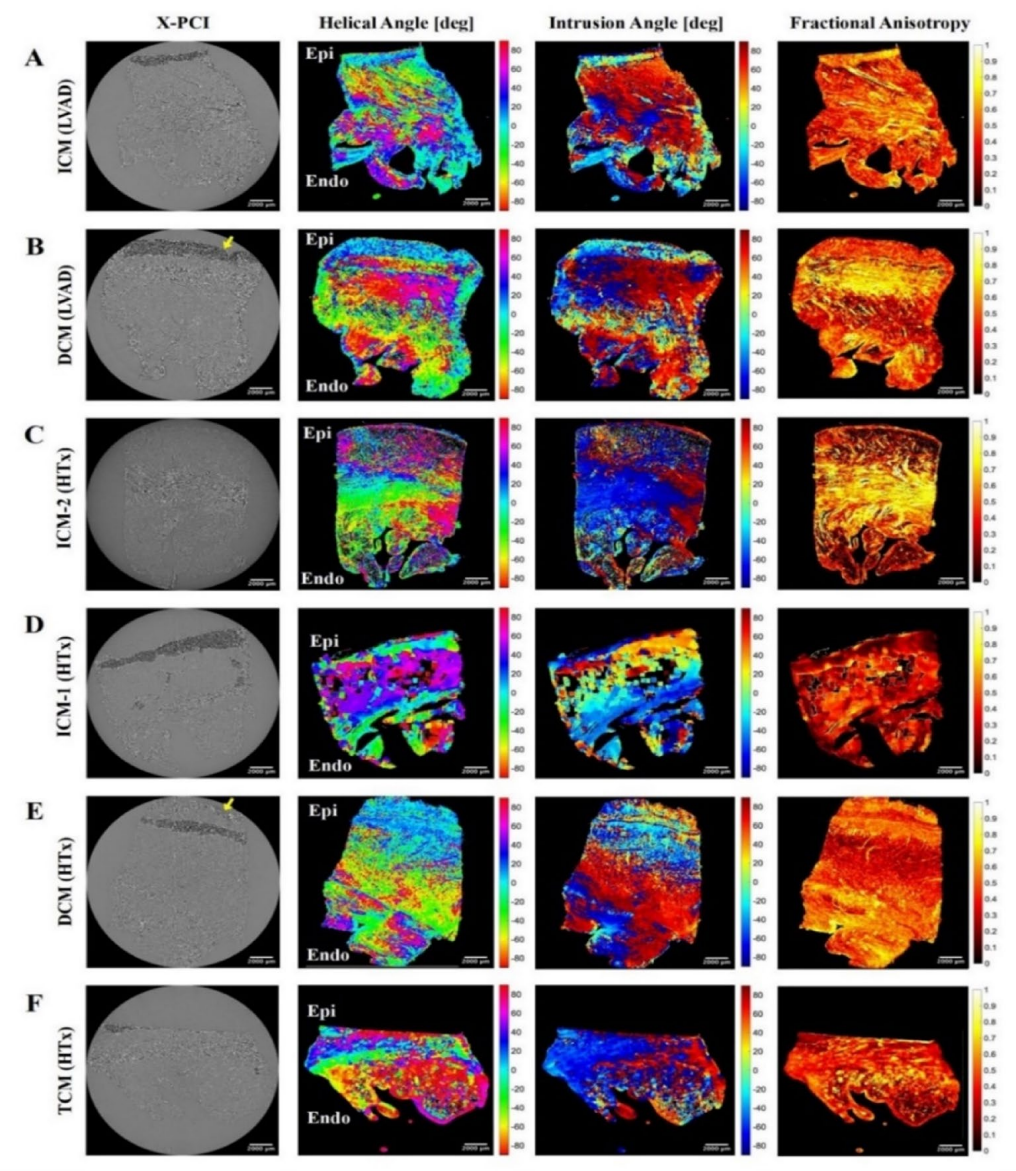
Visualisation of orientation of myocyte aggregates using helical angle (HA), intrusion angle (IA) and fractional anisotropy (FA). The epicardium and endocardium have been labelled as epi and endo, respectively. Epicardial adipose layer was thicker in DCM samples with a thinner epicardial myocyte layer (indicated by yellow arrows). *DCM* dilated cardiomyopathy, *ICM* ischaemic cardiomyopathy, *TCM* toxic cardiomyopathy, *LVAD* left ventricular assist device, *HTx* heart transplantation, *X-PCI* X-ray phase contrast imaging.
